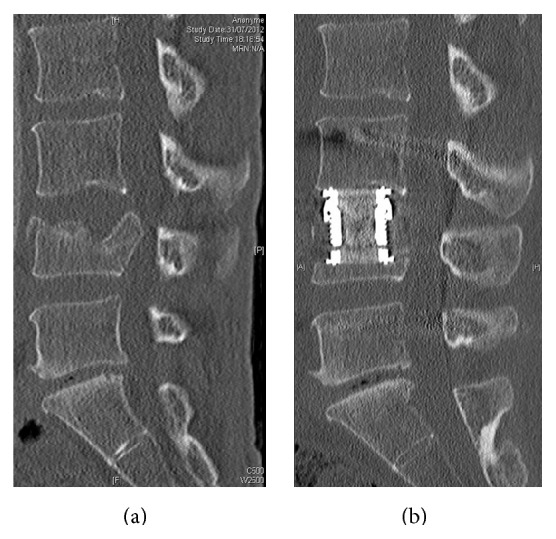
Sagittal pre- (a) and postoperative (b) CT-scan showing a vertebral fracture with a lesion of the superior disc and results after posterior percutaneous osteosynthesis and partial corpectomy.
